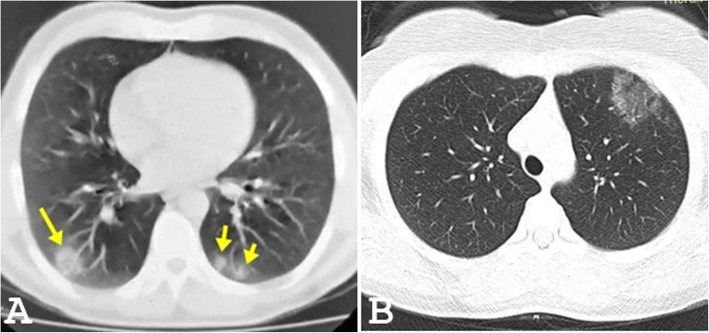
Pulmonary CT findings of COVID-19 patients. a An axial CT image in a 29-year-old patient shows few bilateral peripheral pulmonary nodules 3 days after cough. b An axial CT image in a 33-year-old patient shows single LT upper lobe peripheral ground-glass opacity, 5 days after the onset of fever
